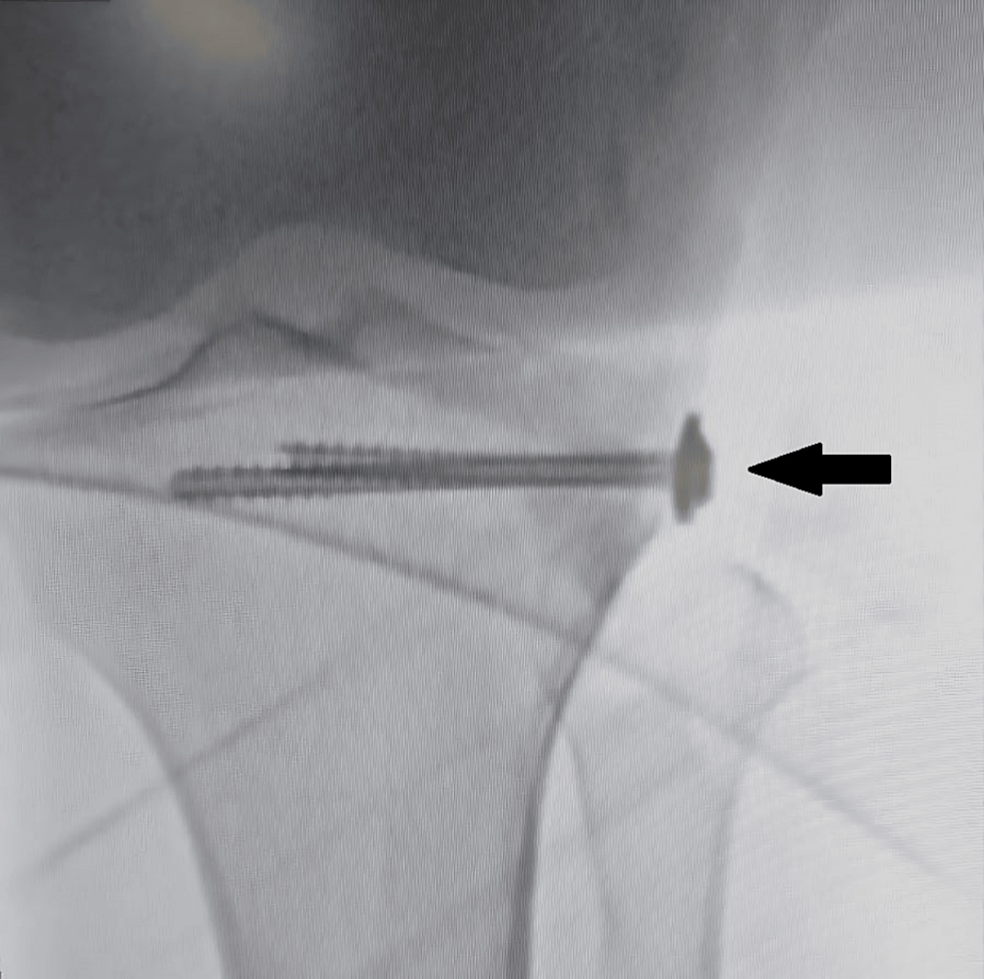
Postoperative X-ray (anteroposterior view) showing void filled with cement and two screws for fixation (black arrow)
